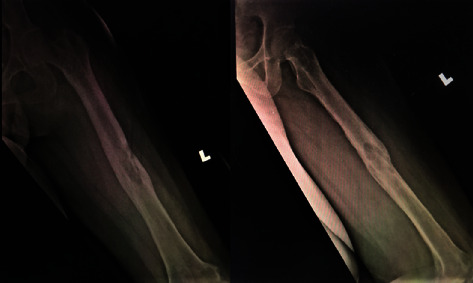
X-rays left femur at 6 months follow-up.
